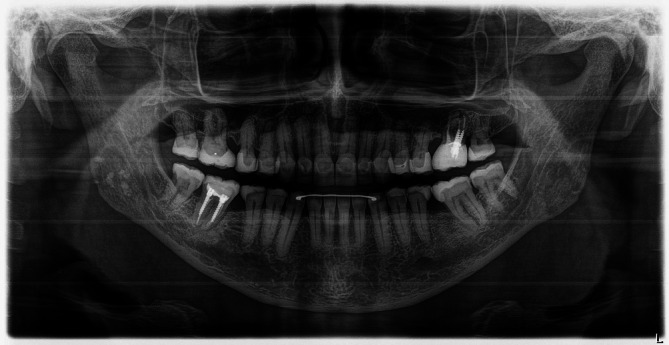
Panoramic radiograph following a clinical follow‐up period of over 11 years.
